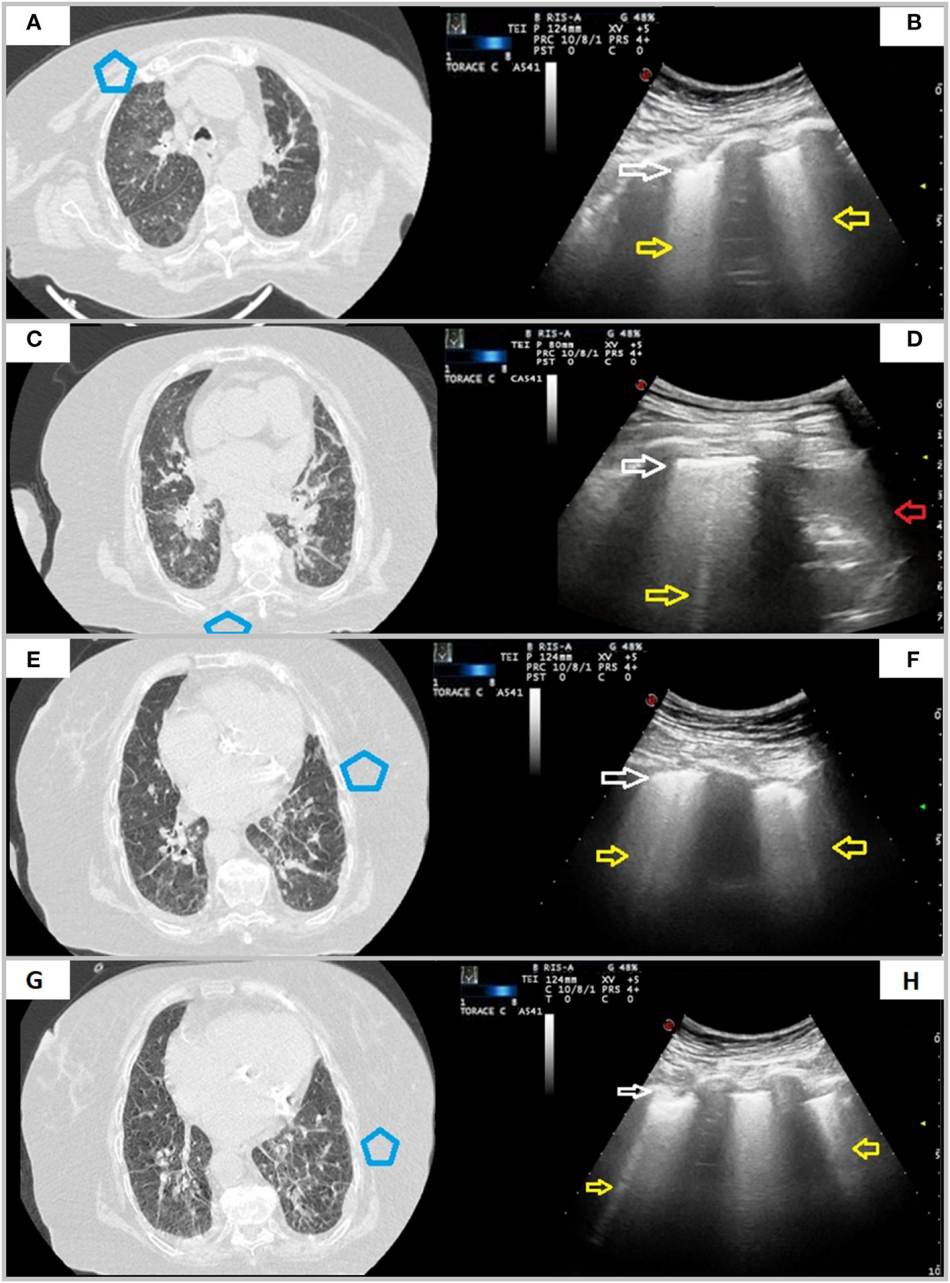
A case of overlapping cardiogenic pulmonary edema. A 76-year-old female patient presenting in ED with dyspnea for 6 days. The RT-PCR assay on nasopharyngeal swab resulted positive for SARS-CoV-2 infection. In (A,C,E,G), axial CT scans reveal smooth interlobular septal thickening, fissural thickening, mixed GGO and consolidation with central distribution, increased artery to bronchus ratio, cardiomegaly, and bilateral pleural effusion. In (B,D,F,H), ultrasound scans with a convex probe (6 MHz) and thoracic setting, corresponding to the blue boxes in the respective CT scans, show an irregular and thickened hyperechoic pleural line (white arrow) followed by focal or coalescent B-lines (yellow arrows). Right posterior ultrasound scan in (H) also underlines presence of pleural effusion (red arrow).
